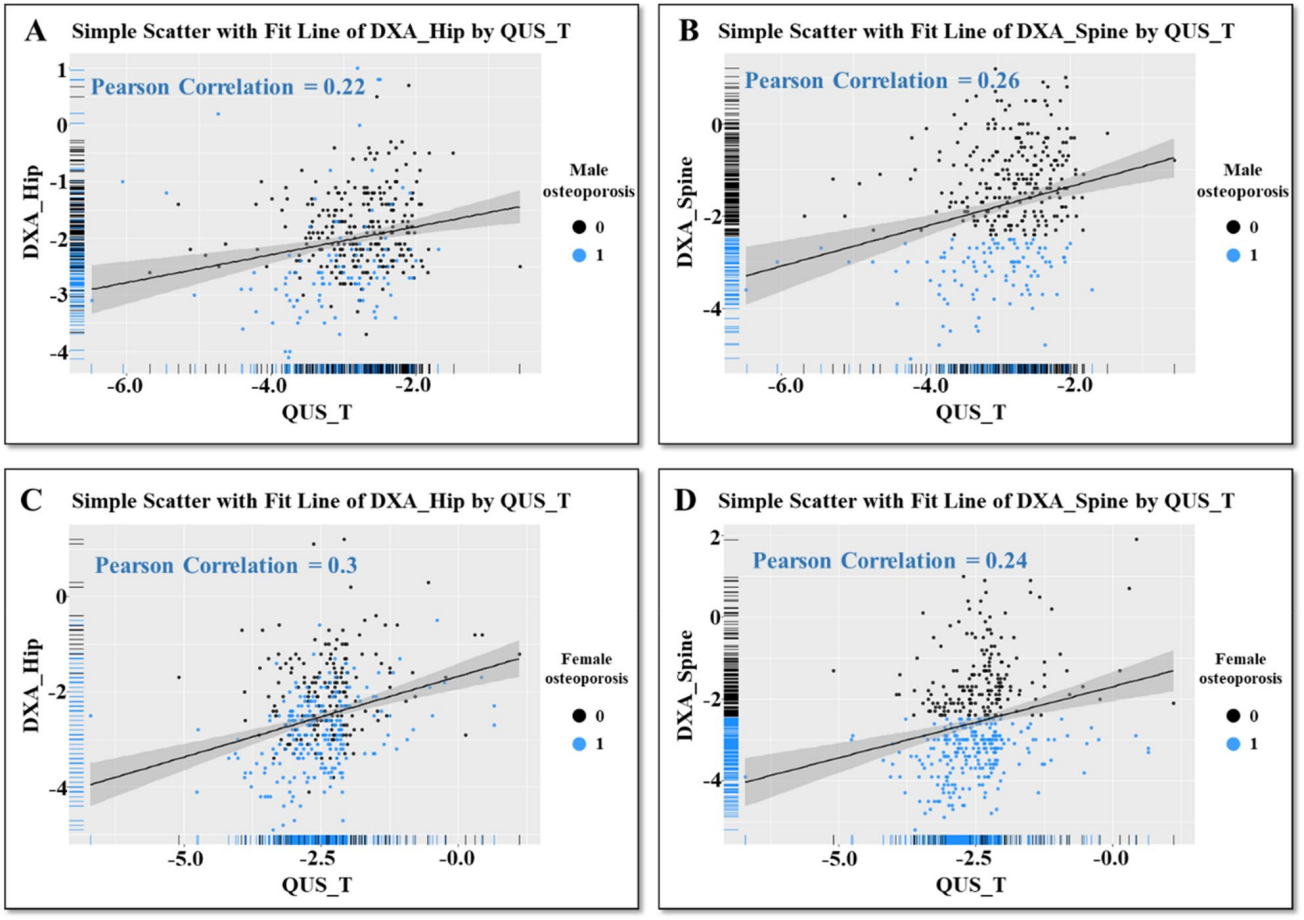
(A) Scatter plot and regression line of calcaneus QUS_T and DXA-Hip of male osteoporosis. (B) Scatter plot and regression line of calcaneus QUS_T and DXA-spine of male osteoporosis. (C) Scatter plot and regression line of calcaneus QUS_T and DXA-Hip of female osteoporosis. (D) Scatter plot and regression line of calcaneus QUS_T and DXA-spine of female osteoporosis. Figure linework and aesthetics were created in R 3.6.2; link to software homepage. *QUS_T* quantitative ultrasonography T-score, *DXA-Hip* dual-energy X-ray absorptiometry of Hip, *DXA-Spine* dual-energy X-ray absorptiometry of Spine.
